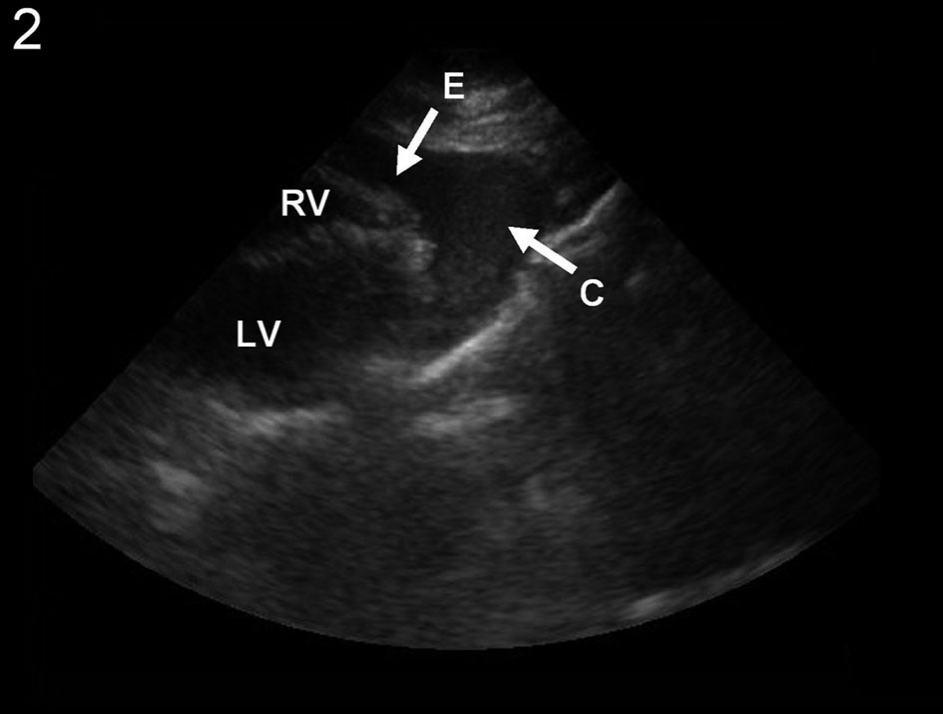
Emergent bedside echocardiogram in the subcostal projection, showing the presence of a moderate pericardial effusion (E), along with clot (C) suggesting hemopericardium. RV: right ventricle; LV: left ventricle.
